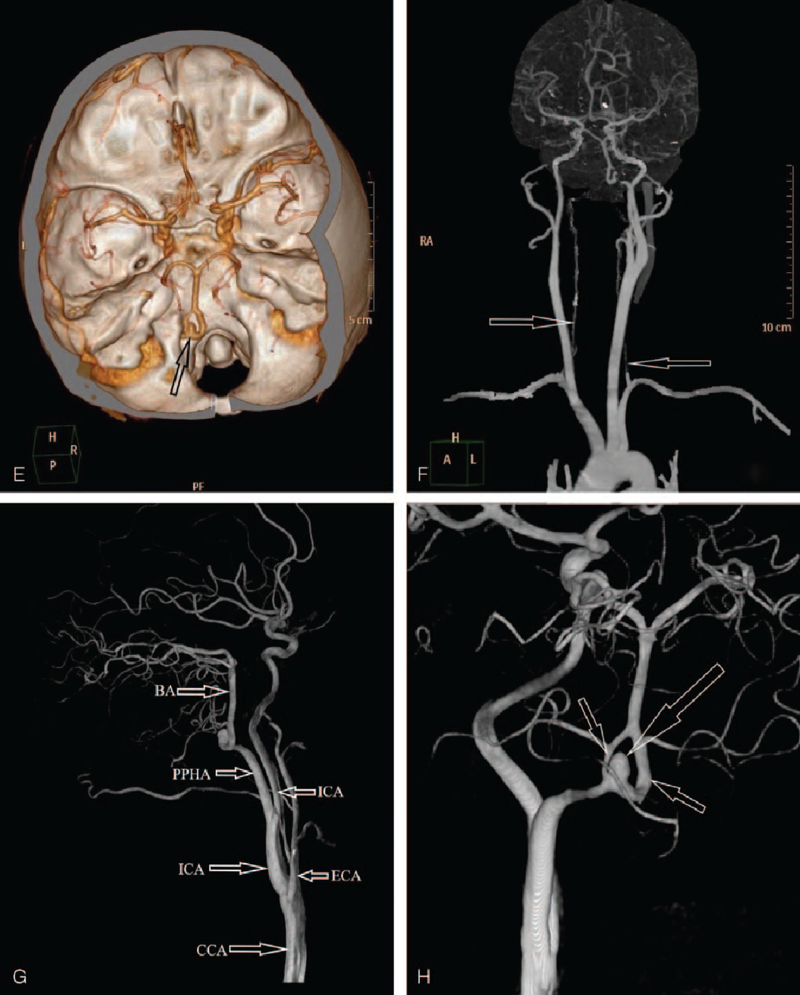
A–D: Are preoperative CT scan, images showing hemorrhage and a PPHA. A: Is a CT scan showing fourth ventricular hemorrhage. B: Is a CT scan showing third ventricular and bilateral ventricular hemorrhages. C: Is a CT scan showing a PPHA originating from the internal carotid artery (ICA) at the plane of the C1 and C2 vertebral bodies. D: Is a CT scan showing a PPHA entering the skull through the hypoglossal canal. E–H: Are preoperative CTA and DSA images showing a fenestrated malformation with a cystic protrusion on the lower left side. E: Is a CTA showing a fenestration of the PPHA after entering the skull with a ruptured aneurysm. The bilateral posterior communicating arteries are absent. F: Is a DSA image showing very tine bilateral vertebral arteries throughout their course and do not provide blood supply to the basilar artery. G: Is a DSA image showing the posterior circulation. The blood supply of PPHA comes from the ipsilateral internal carotid artery. H: Is a DSA image showing a PPHA, an aneurysm and a fenestrated malformation. CT = computerized tomography, CTA = computed tomographic angiography, DSA = digital subtraction angiography, PPHA = persistent primitive hypoglossal artery.
